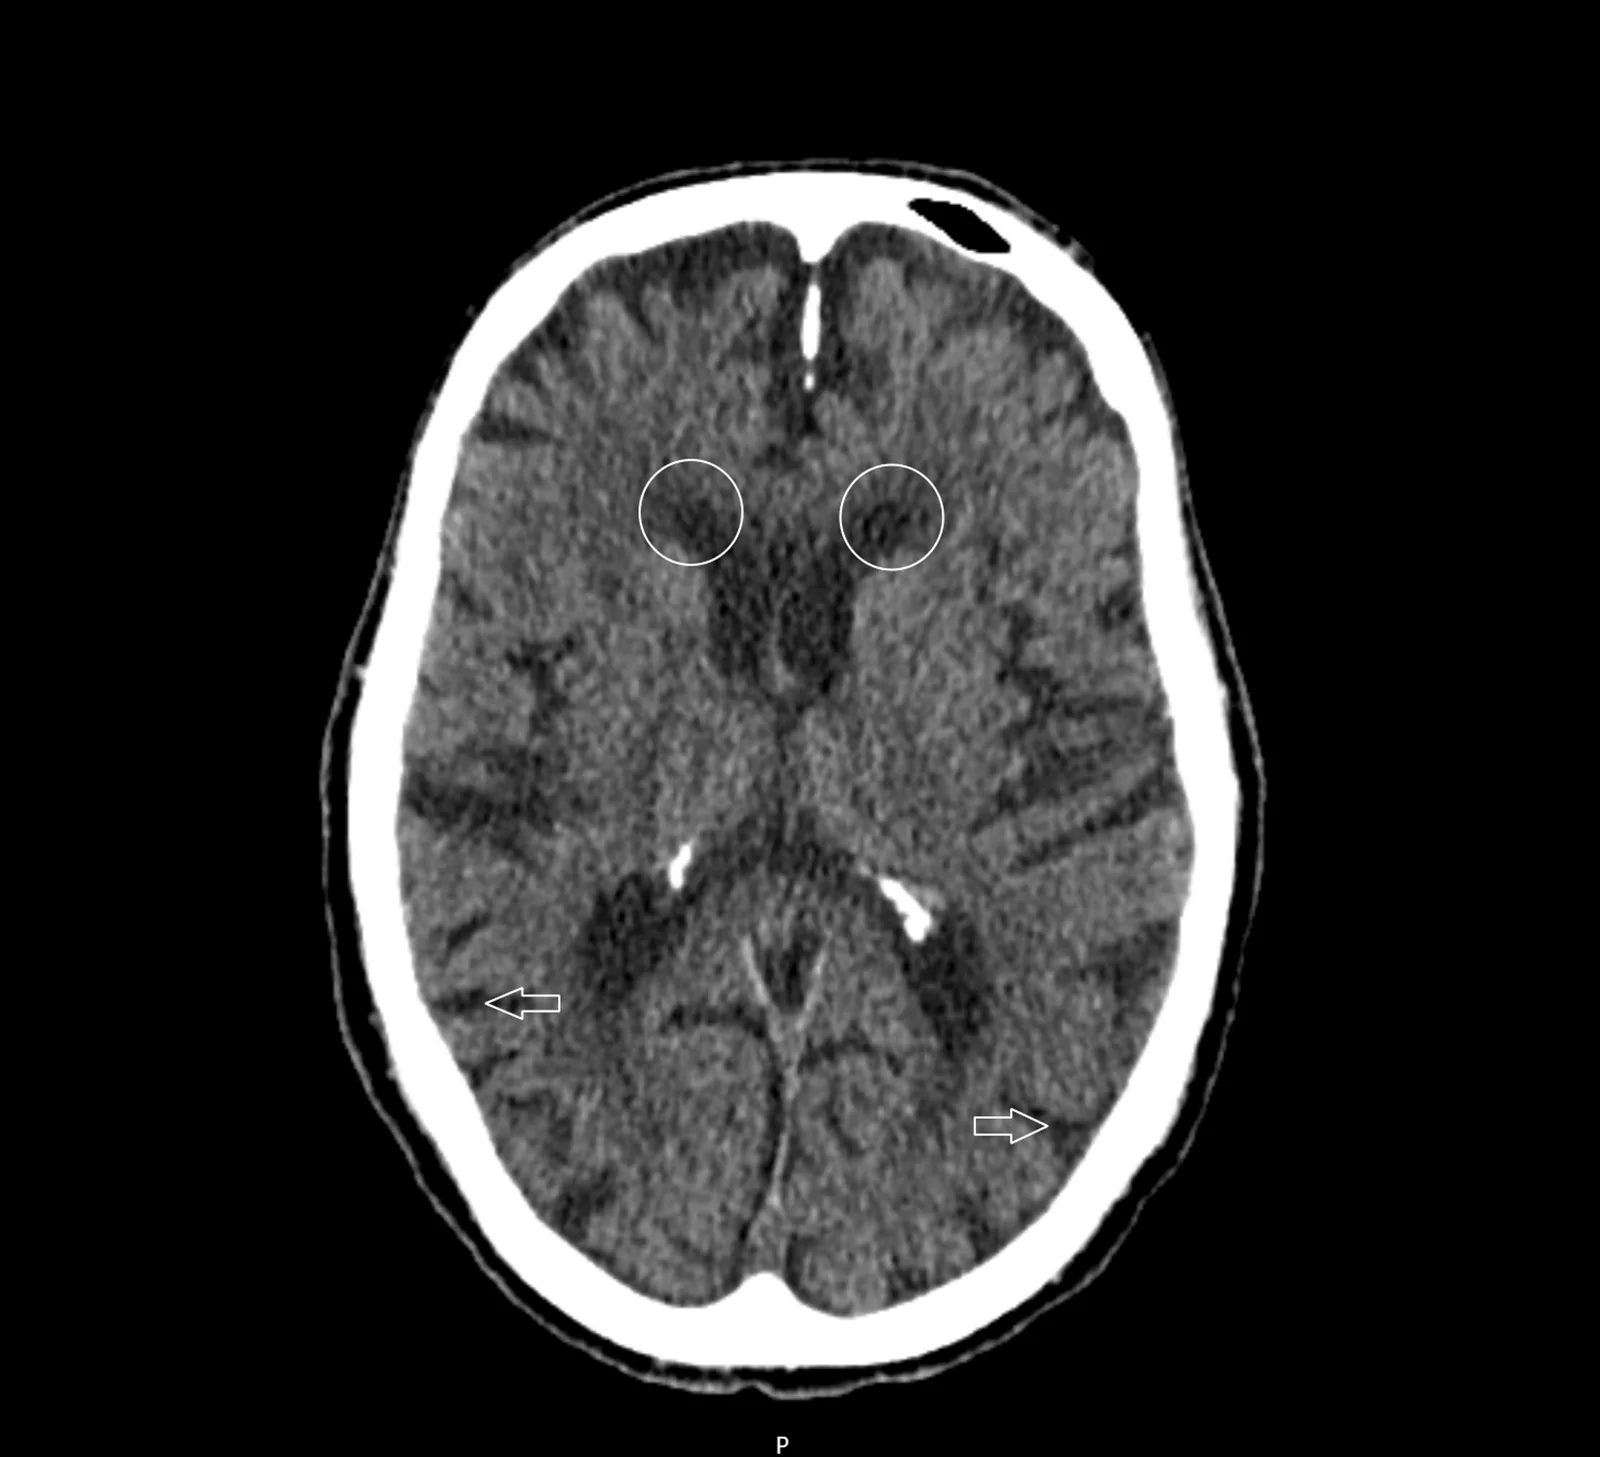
Non-contrast cranial computed tomography. Axial non-contrast cranial computed tomography showing bilateral periventricular hypodensities (open circles), compatible with chronic ischemic leukoencephalopathy, and prominent cortical sulci (arrows), consistent with cerebral atrophy. No acute intracranial abnormality is identified.
